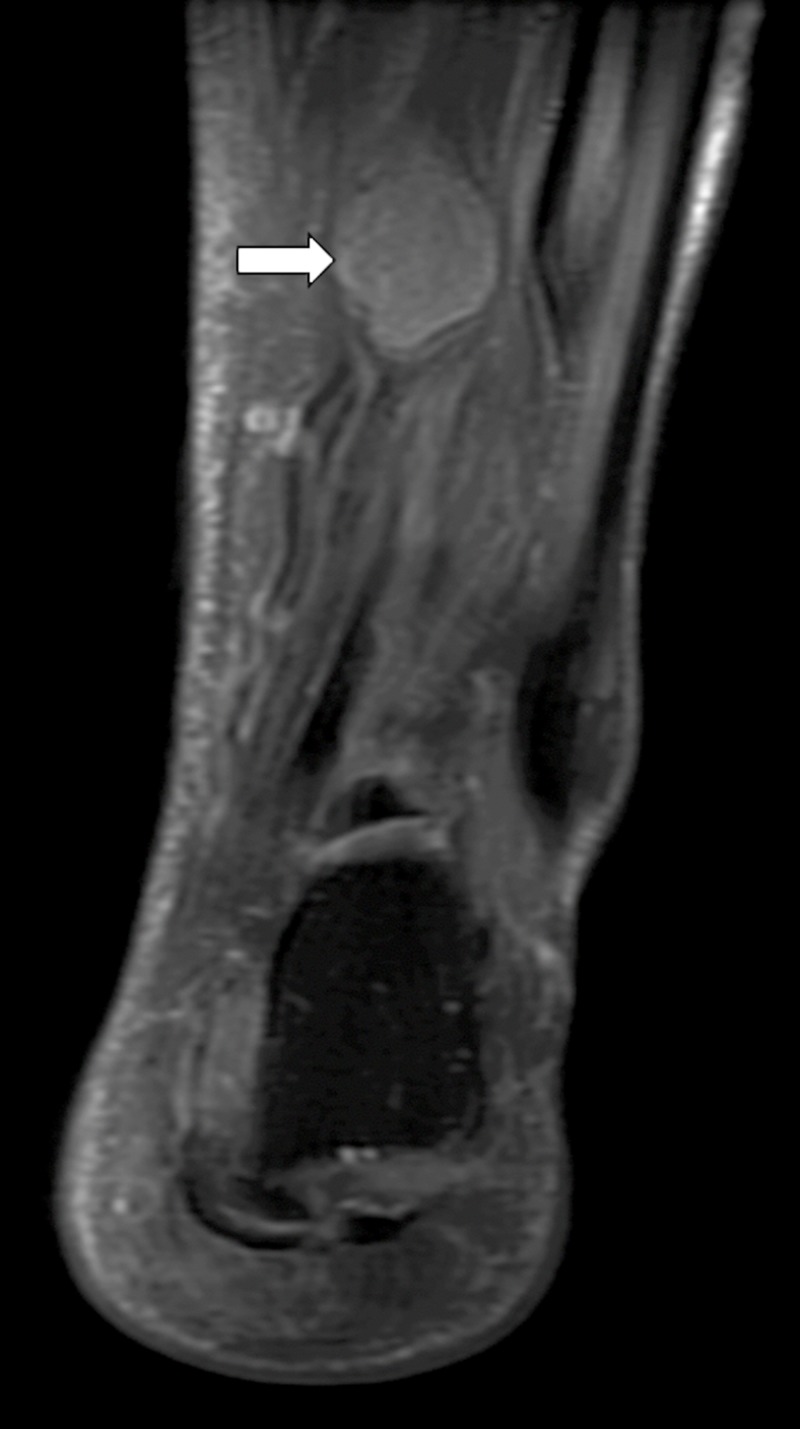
T2 weighted coronal MRI showing the mass arising from posterior tibial nerve
